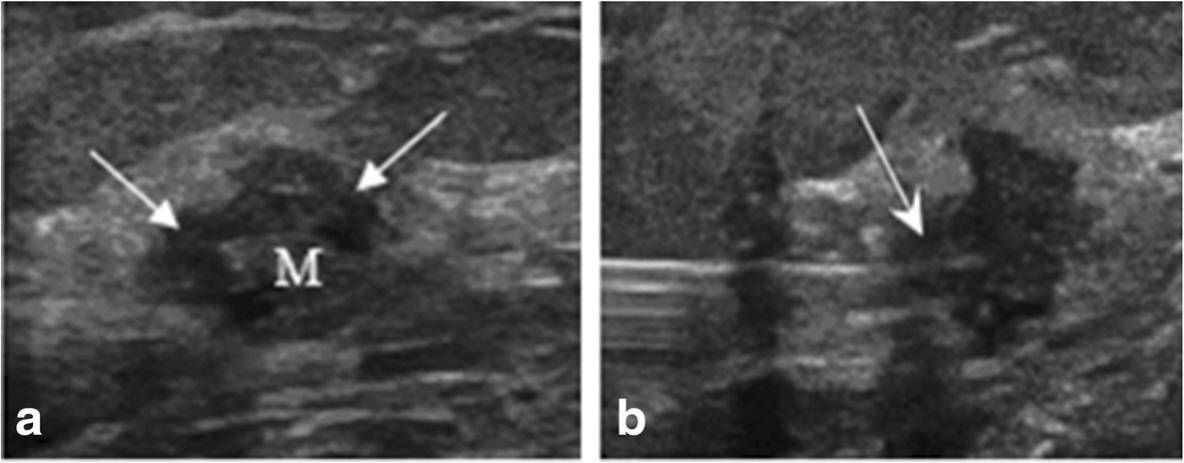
Ultrasonographic appearance of a nonpalpable breast lesion: a hypoechoic with irregular margins; b intralesional injection of indocyanine green (ICG) solution with a spinal needle under ultrasound guidance (*arrow*)
